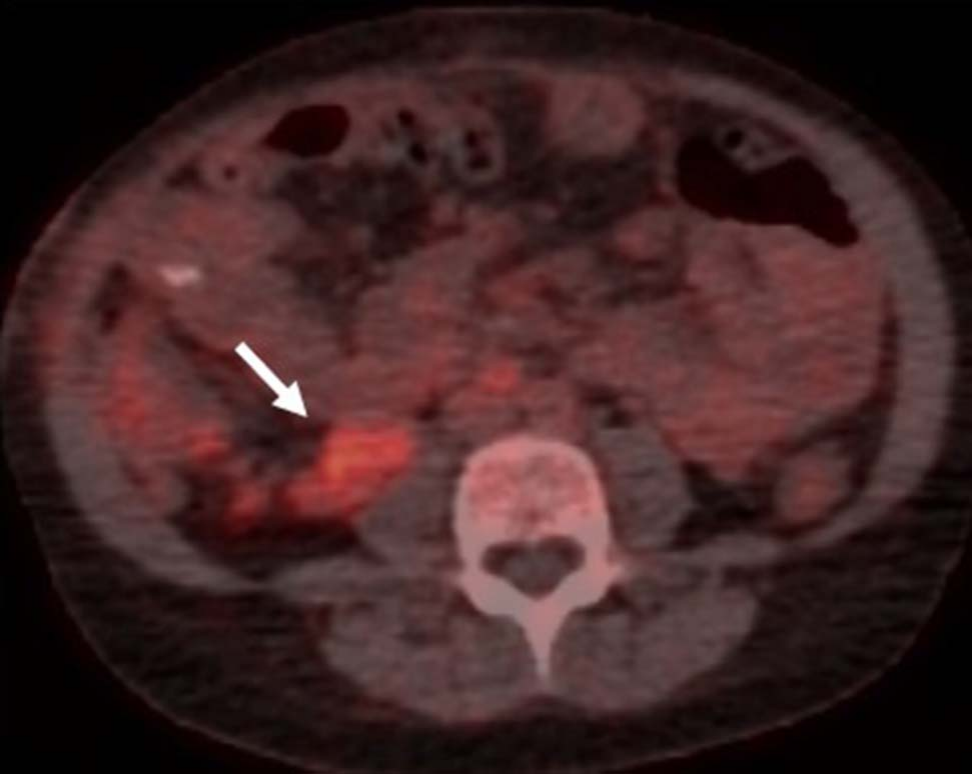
FDG PET/CT scan demonstrated increased metabolic activity of the retroperitoneal (SUV 4.7) lesions seen on contrast CT images. CT, computed tomography; PET, positron emission tomography; SUV, standardized uptake value.
